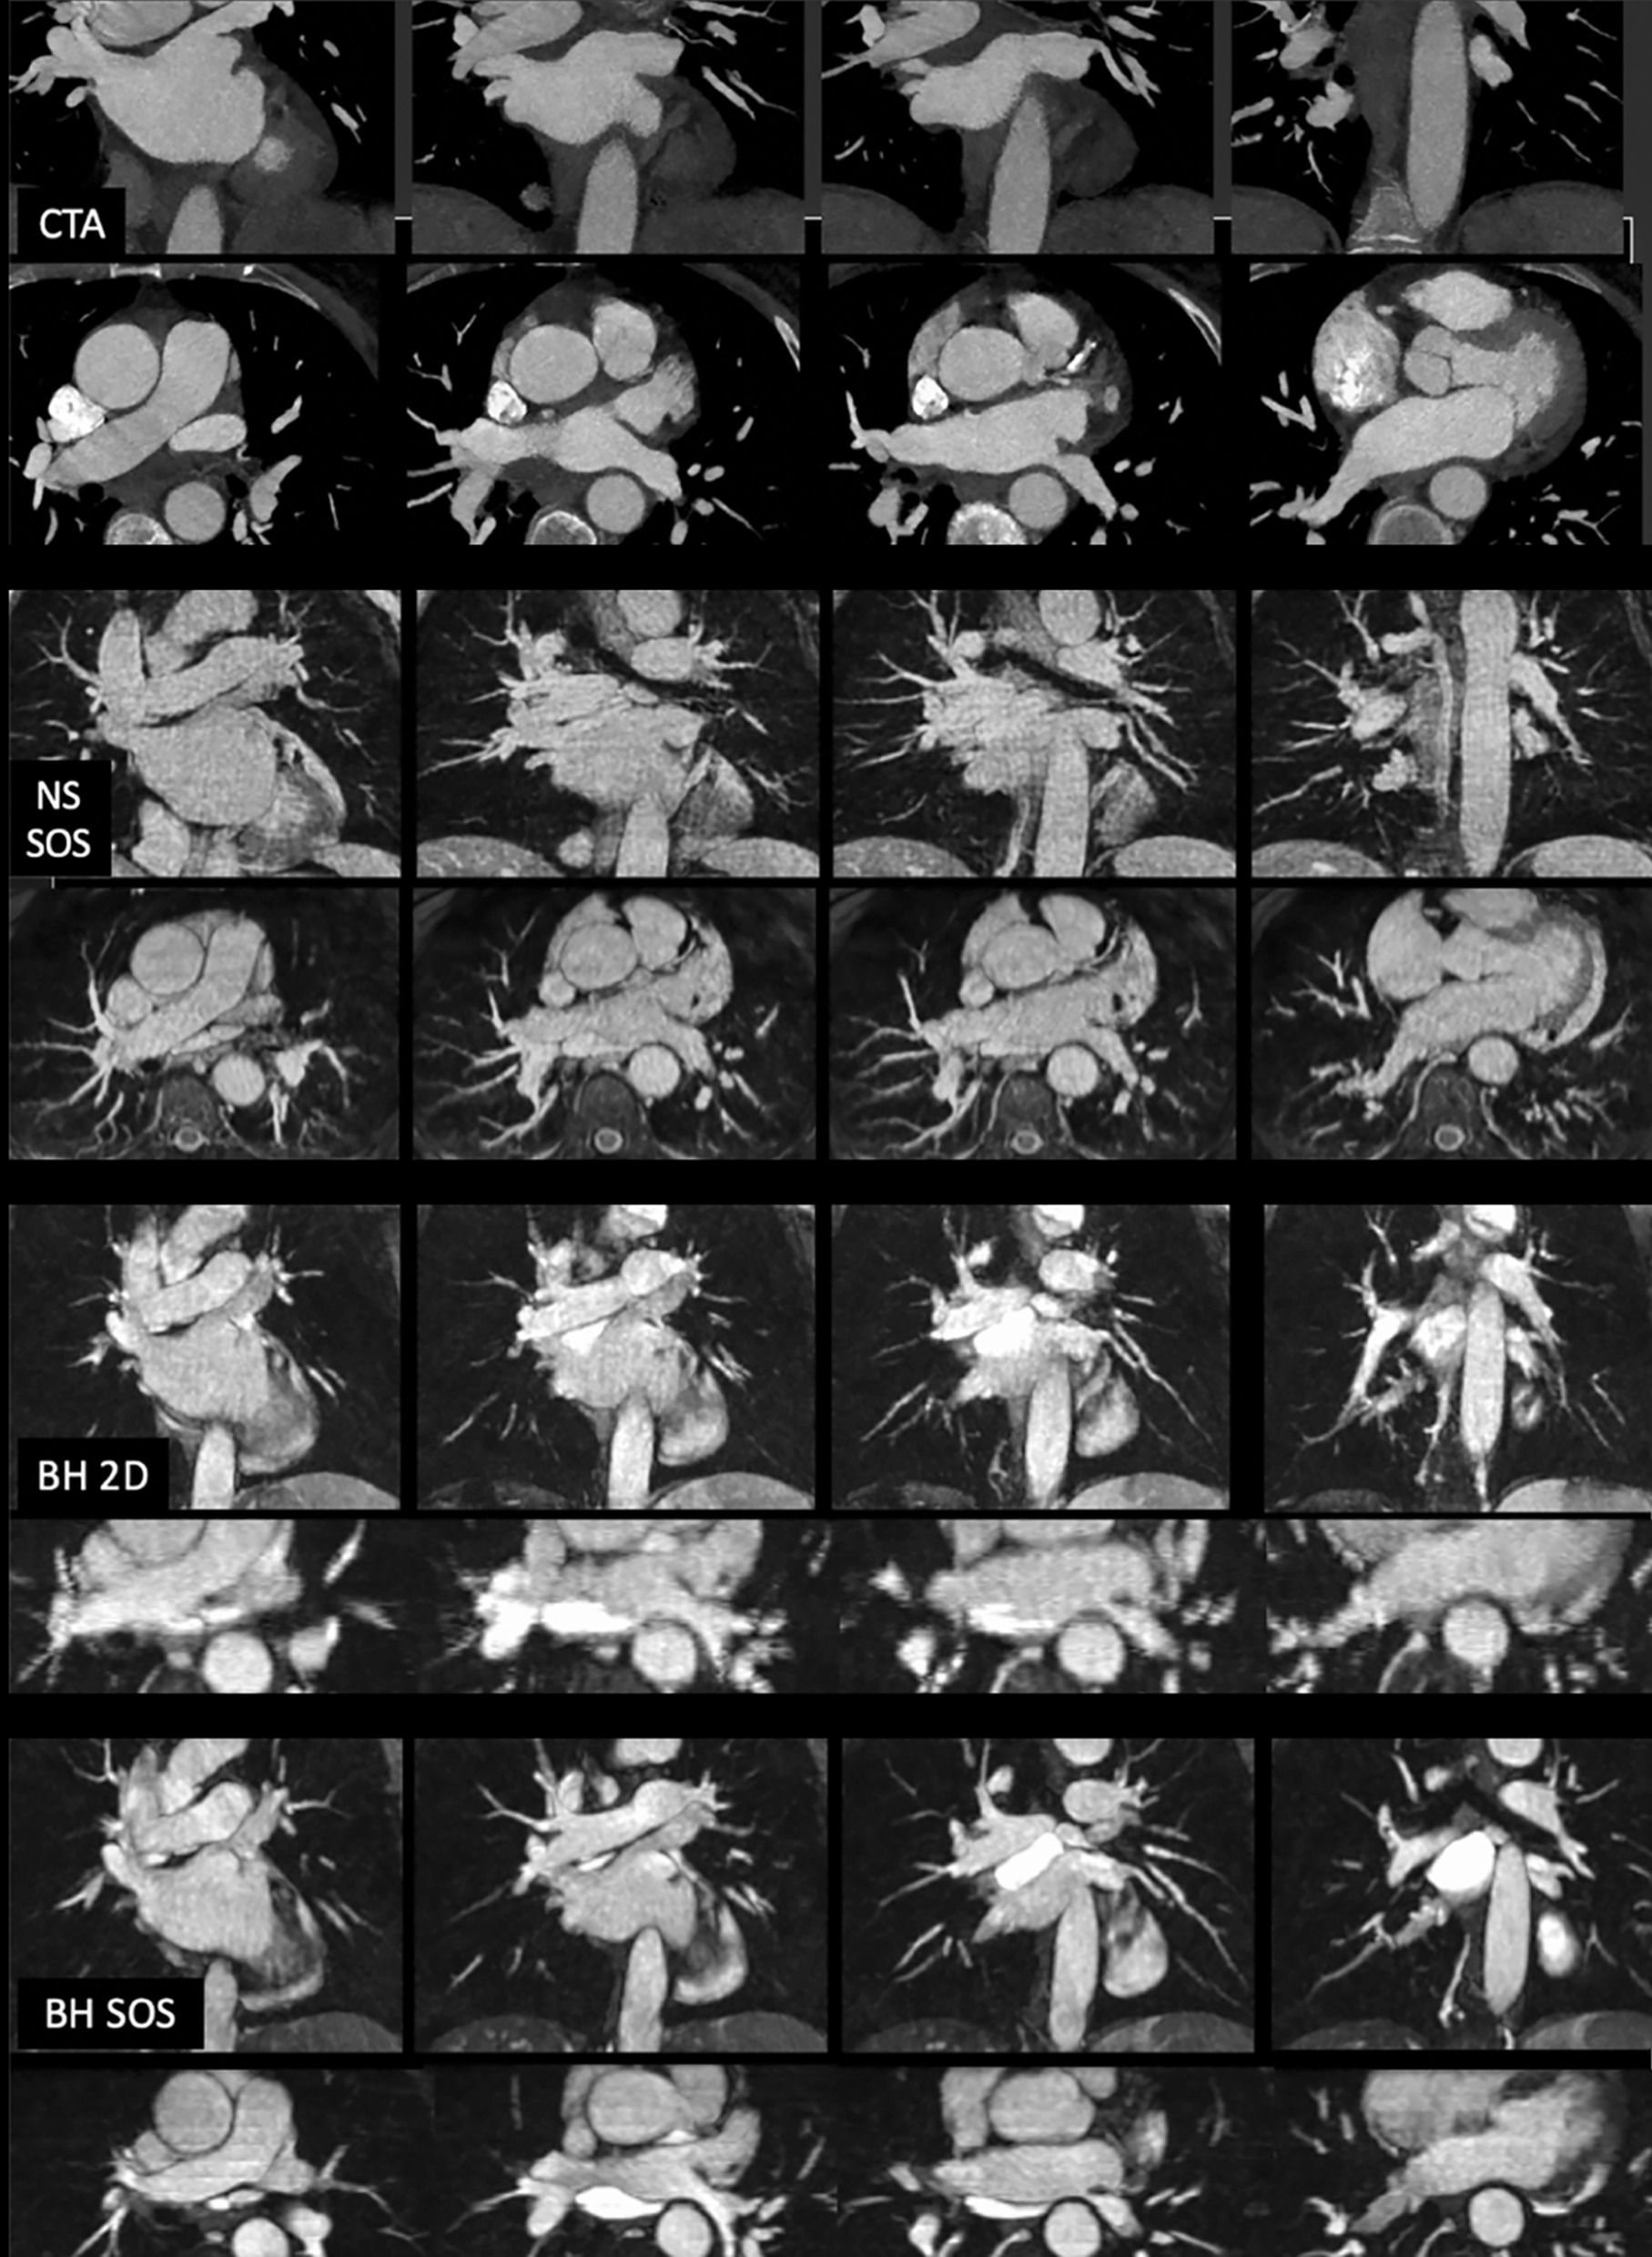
Comparison of maximum intensity projections from coronal and axial computed tomography angiography (CTA), navigator-gated free-breathing 3D stack-of-star bSSFP using a spatially non-selective RF excitation (NS-SOS), breath-hold 2D radial bSSFP (BH-2D) and breath-hold 3D stack-of-stars bSSFP (BH-SOS). For NS-SOS, BH-2D and BH-SOS, both axial and coronal maximum intensity projections were reconstructed from a coronal scan. All three non-contrast CMRA techniques show good image quality in the coronal maximum intensity projections. However, more vascular blurring is apparent in the BH-2D axial maximum intensity projections due to the larger slice thickness and worse slice profile compared with the 3D acquisitions
